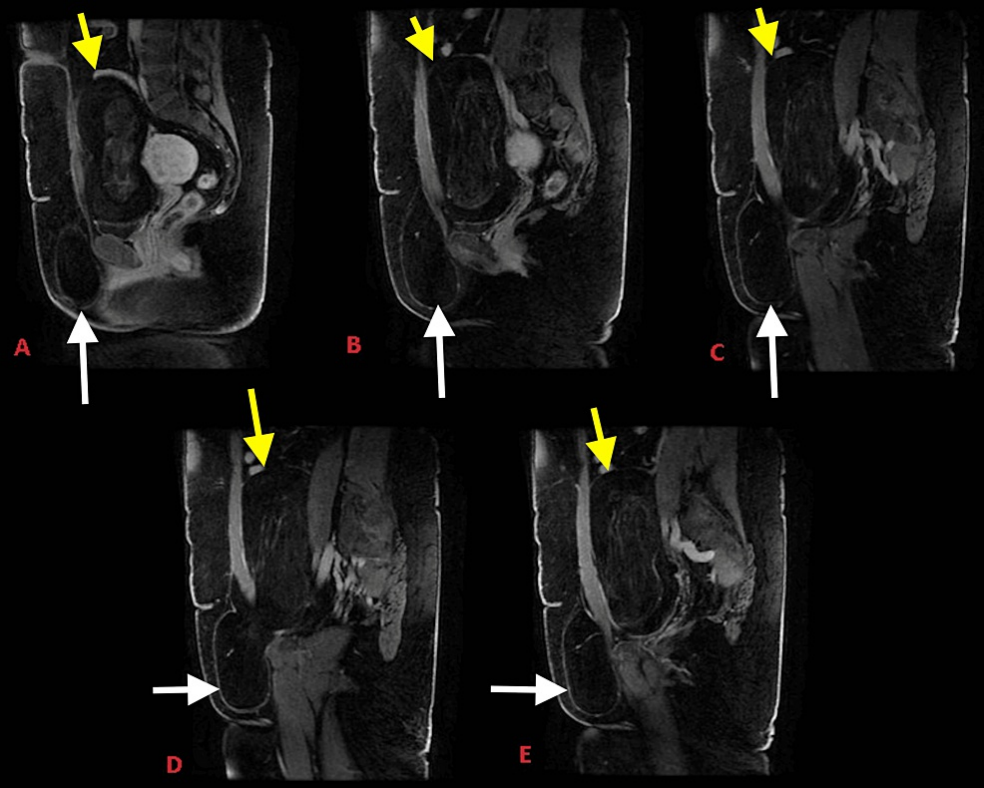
Abdomen and pelvis MRI. Sequential sagittal sections in the contrast-enhanced phase. White arrows point to the extra-abdominal component of the lipoma and yellow arrows point to the intra-abdominal component of the lipoma Abdomen and pelvis MRI. Sequential sagittal sections in the contrast-enhanced phase: lesion with intra- and extra-abdominal components, hourglass-shaped, narrowing at the passage through the inguinal ring in the abdominal wall. Measures 20 x 11 x 11 cm in the largest diameters (longitudinal x lateral x anteroposterior), at the inguinal ring passage, the "hernia" neck measures 4.1 x 4.4 cm (lateral x longitudinal). Within the lesion, bands of edema are observed, which are enhanced with intravenous gadolinium, while the remainder shows no enhancement. The lesion's volume displaces the midline of the genitalia to the right, compresses and displaces the uterus and bladder to the right inside the abdomen, separates adjacent intestinal loops, compresses and bulges anteriorly the wall of the left hemiabdomen in the hypogastrium, compresses and laterally displaces the left iliac vessels, and has intimate contact with the left iliopsoas muscle.
